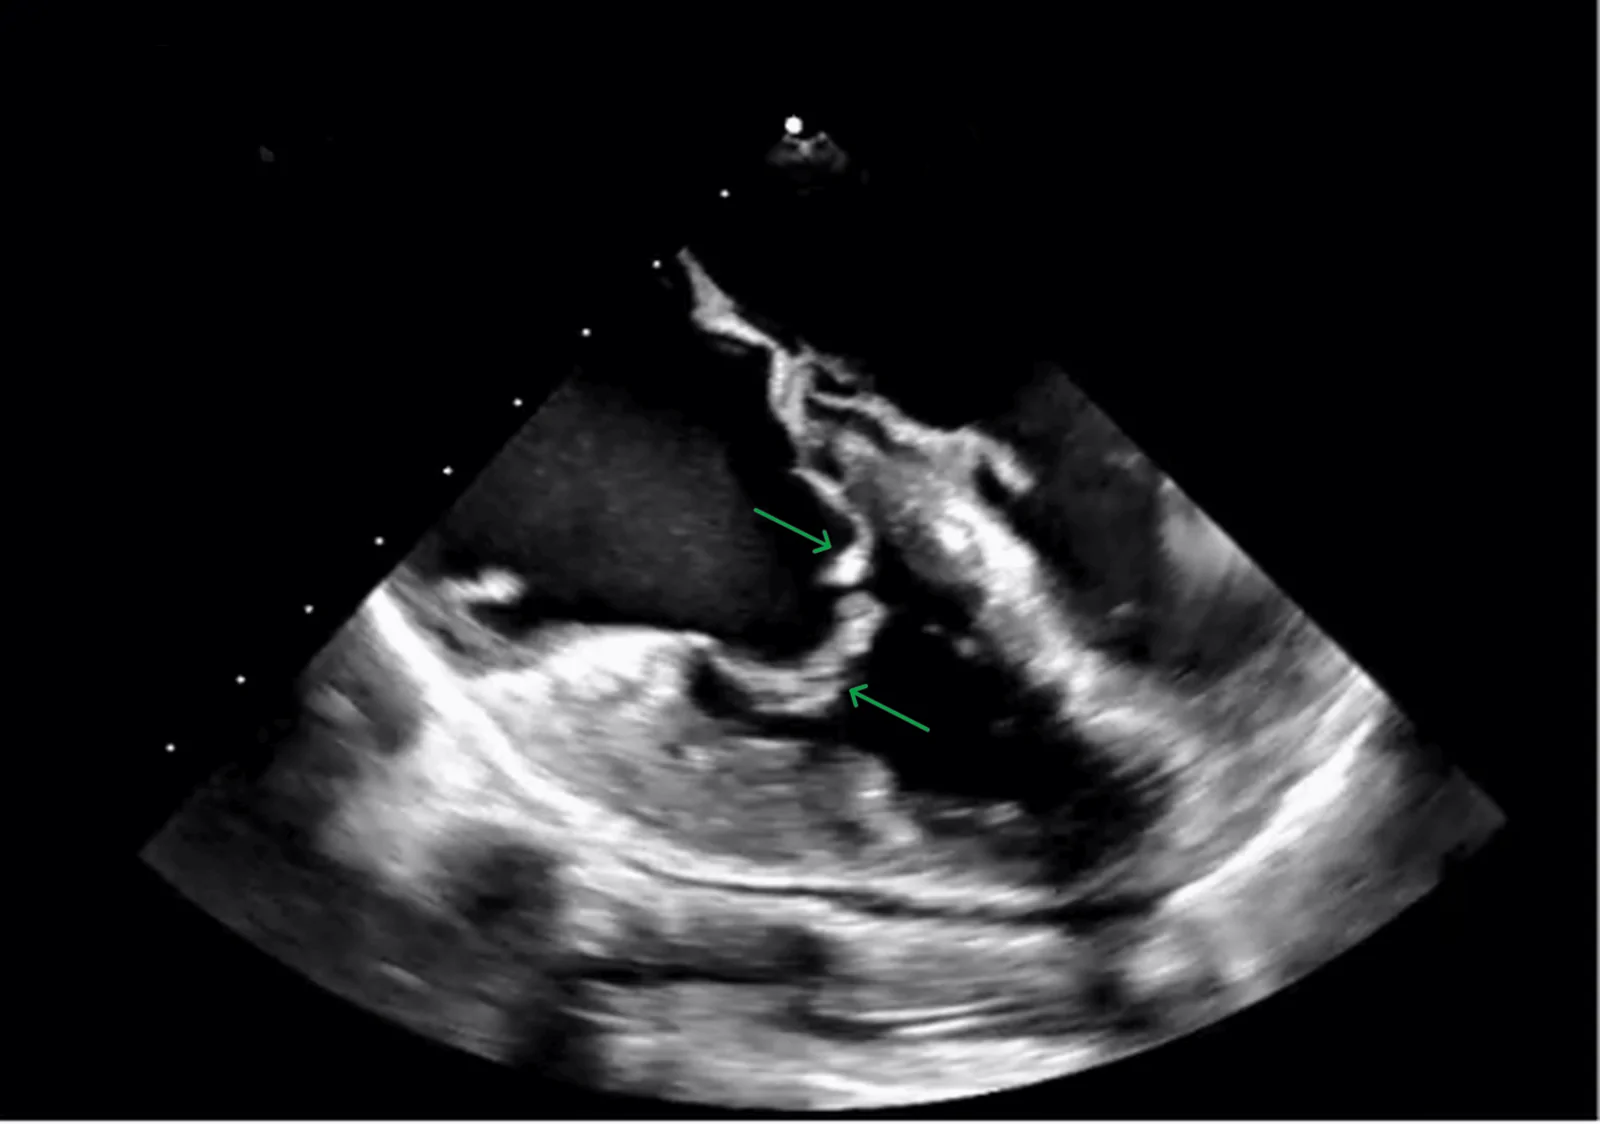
Transoesophageal echocardiogram (TOE) image showing thickening of the tricuspid valve leaflets (green arrows) (mid-oesophageal four chamber view).
